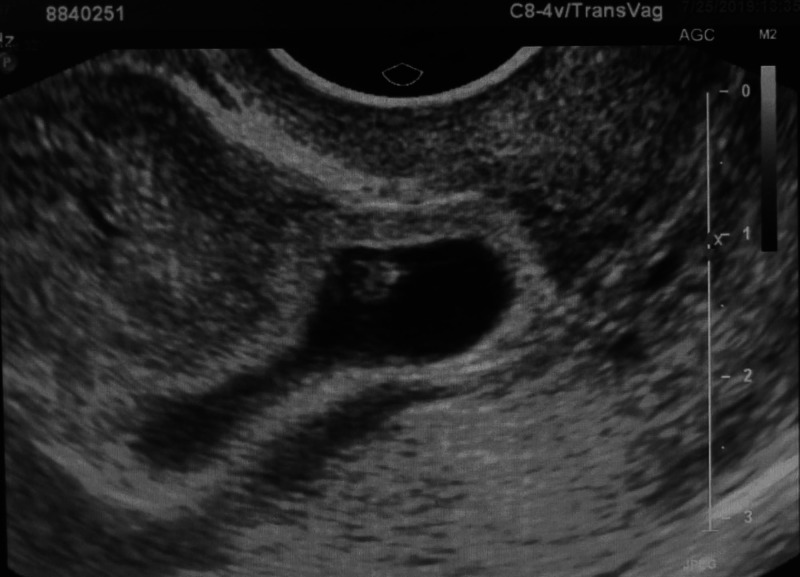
Transvaginal ultrasound showing cesarean scar ectopic pregnancy at 6 weeks and 3 days.
